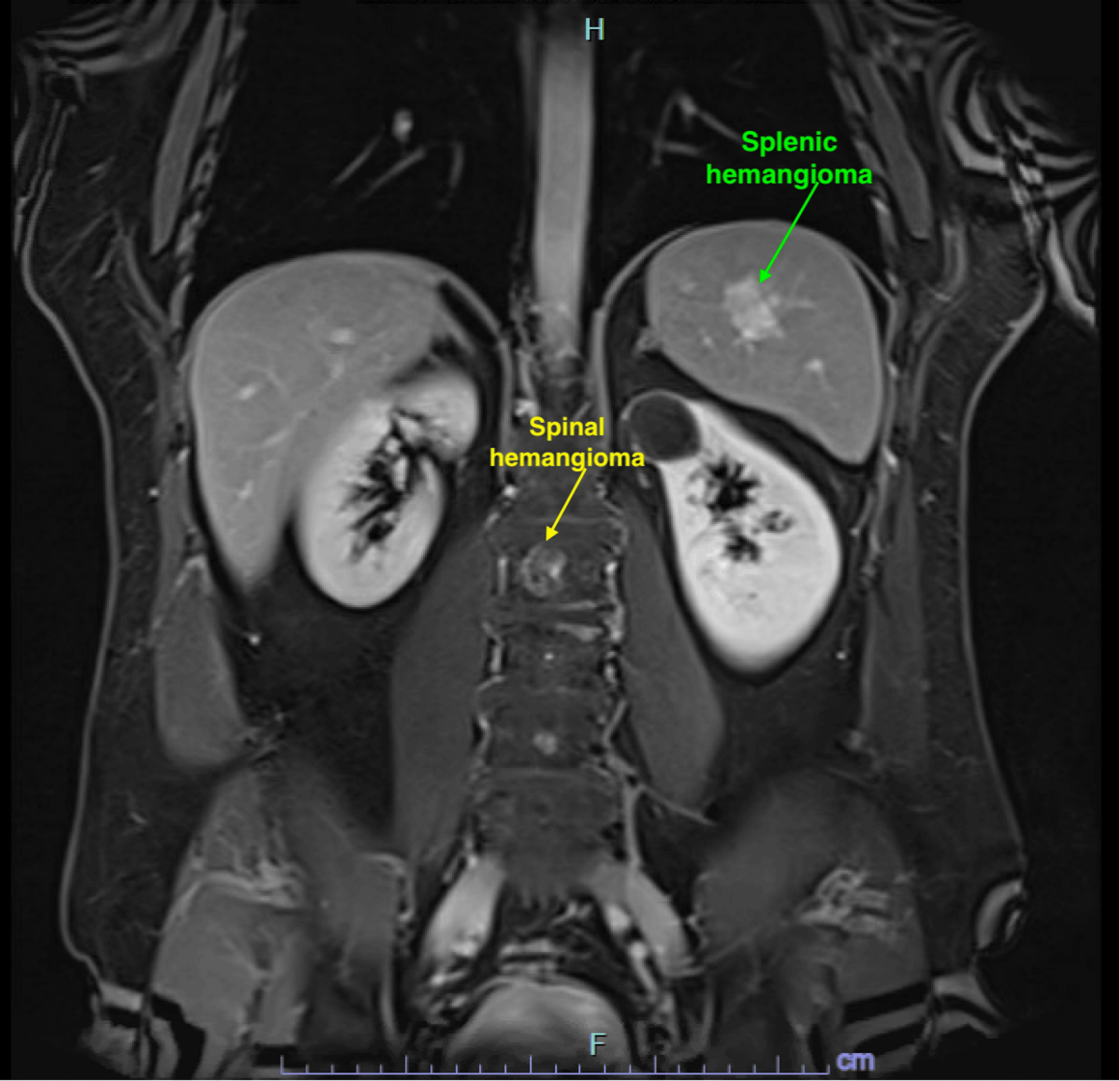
MRI of the abdomen showing splenic hemangioma (green arrow) and spinal hemangioma (yellow arrow). MRI = magnetic resonance imaging
